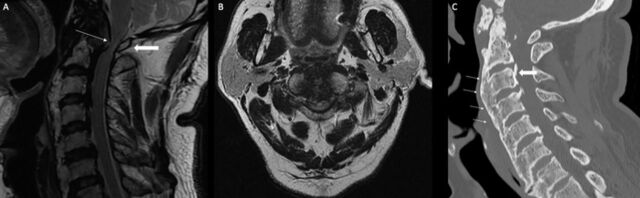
Noncontrast T2-weighted (A) sagittal and (B) axial magnetic resonance imaging of the cervical spine obtained on initial presentation shows significant canal stenosis (block arrow) at the craniovertebral junction secondary to significant ventral soft tissue compression and tectorial membrane buckling (arrow). (C) Noncontrast sagittal computed tomography of the cervical spine shows diffuse idiopathic skeletal hyperostosis with continuous anterior (arrows) and segmental posterior (block arrow) ossified longitudinal ligaments extending into the anterior atlanto-occipital and tectorial membranes.
